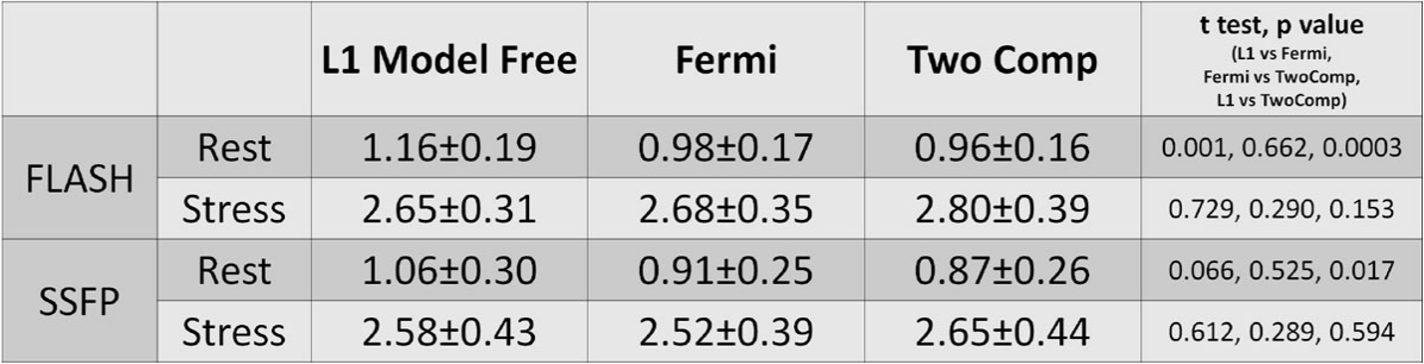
Measured Myocardial Blood Flow values for rest and stress perfusion study. First pass perfusion is used in L1 model free and Fermi doconvolution and the whole data ranged is used in two compartment model. N = 16 patients underwent rest and Adenosine stress perfusion studies with local IRB approval and written consent for either FLASH (N = 8) or SSFP (N = 8) protocols. Imaging experiments were performed on a 3T clinical MRI system (MAGNETOM Skyra, Siemens). 60 heart beats (including 3 PD beats) were imaged with three short-axis slices being acquired per beat. Typical imaging parameters for FLASH were: FOV 360 × 270 mm^2^, 14° flip angle, 8 mm slice thickness, interleaved parallel acceleration R = 3, acquired matrix 192 × 111, single shot imaging duration of 53 ms. SSFP protocol used 50° flip angle and had single shot duration of 67 ms. The Gd dose was 0.075 mmol/kg for FLASH and 0.05 mmol/kg for SSFP administered at 2 ml/s.
